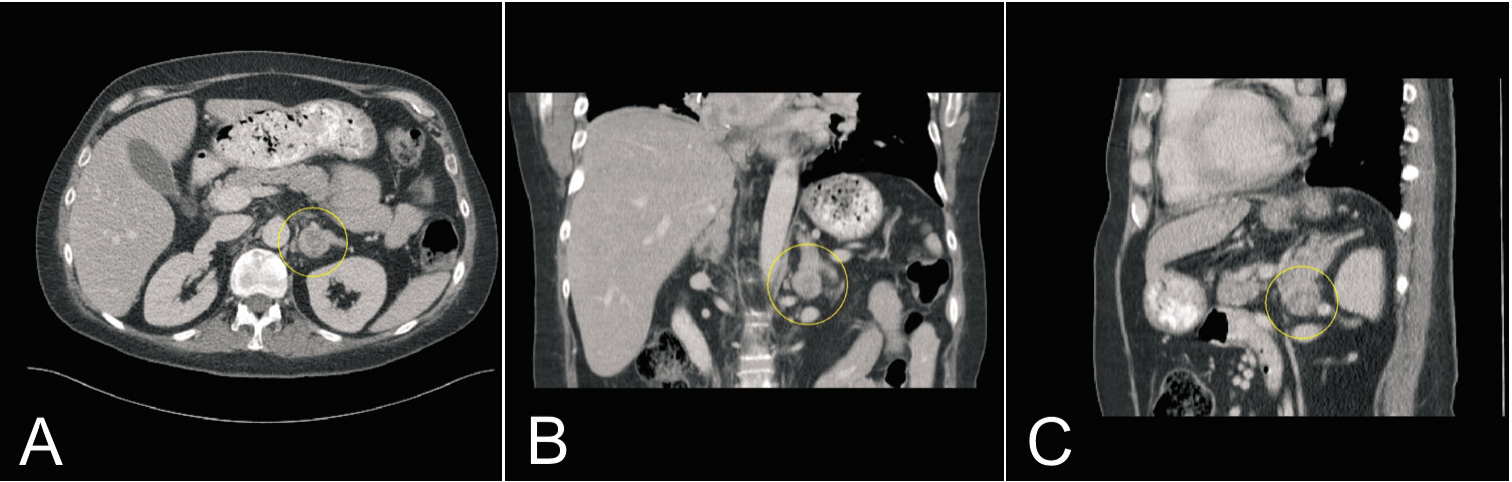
Abdominal CT scan demonstrating the horizontal (a), coronal (b) and sagittal (c) section of a tumor in the left adrenal gland (circle).
